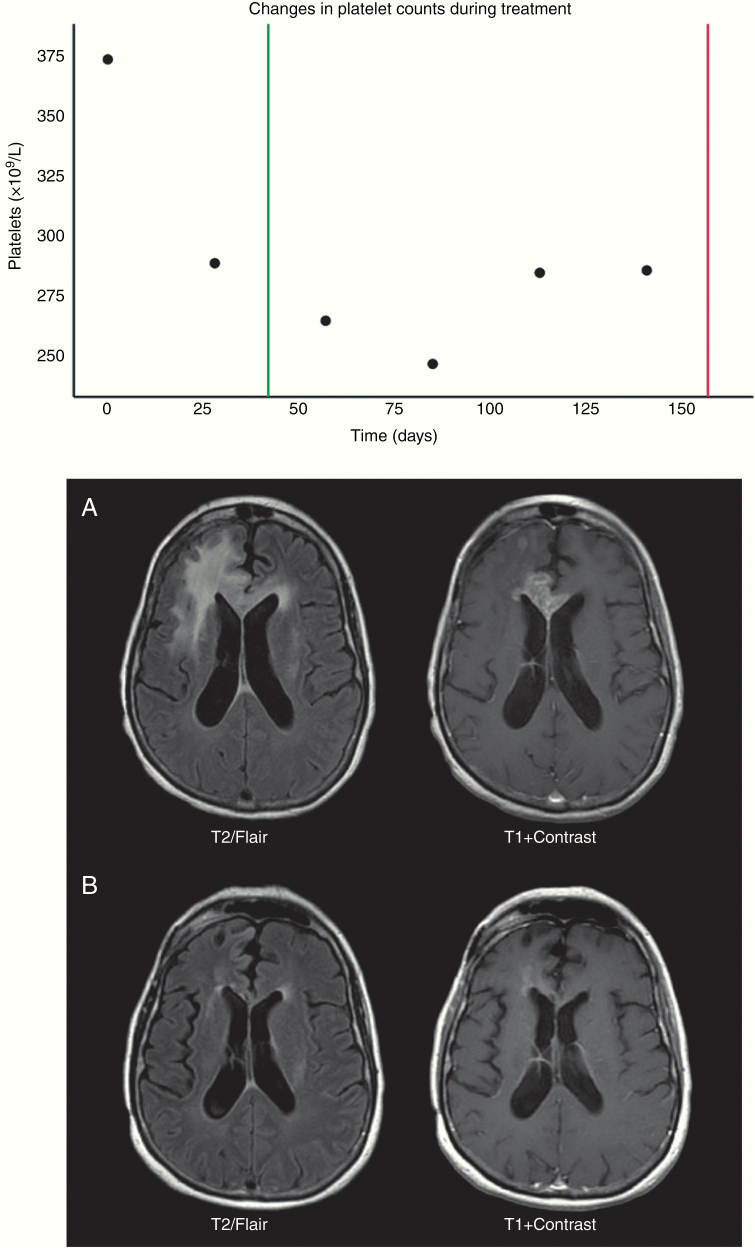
Changes in platelet counts correlate with bevacizumab response. (A) A representative patient’s platelet counts over the duration of bevacizumab therapy. Response and progression dates denoted in green and red, respectively. Platelet count decreases correspond with a response to treatment, with increases preceding evidence of progressive disease. (B) T2/FLAIR and T1-postcontrast images at the time of bevacizumab initiation (a) and at the time of response to bevacizumab (b).
